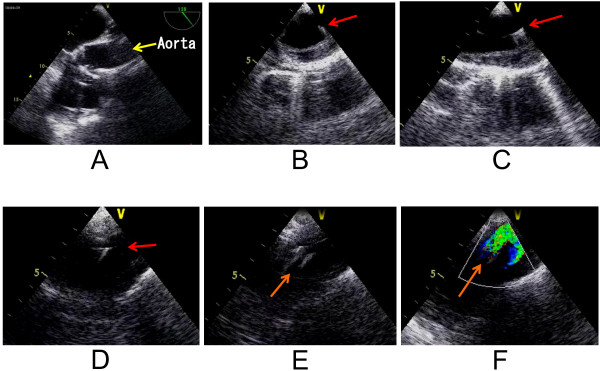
Images of transesophageal echocardiography. (A) Needle insertion is difficult to visualize in the ascending aorta. (B,C,D) Guide wire (arrows) in the true lumen. (B) Short axis of descending thoracic aorta. (C) Long axis of descending thoracic aorta. (D) Distal aortic arch. (E) Cannula tip (arrow) in the distal aortic arch. (F) Color Doppler flow through the cannula forms a U-turn shape. Arrow indicates direction of perfusion through the cannula.
